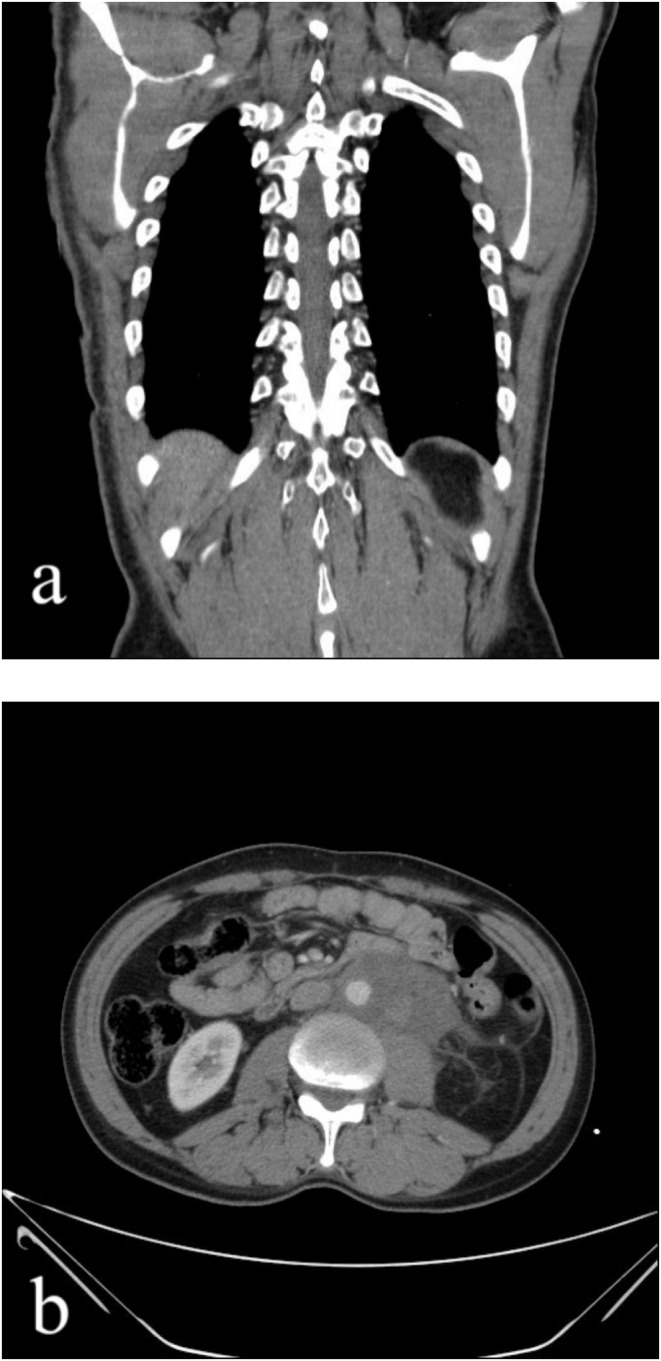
Contrast‐enhanced computed tomography (CT) in coronal view (a) and axial view (b) showing a 14 × 12 × 11 cm soft tissue mass in the left retroperitoneum suggestive of a metastatic tumoral mass.
